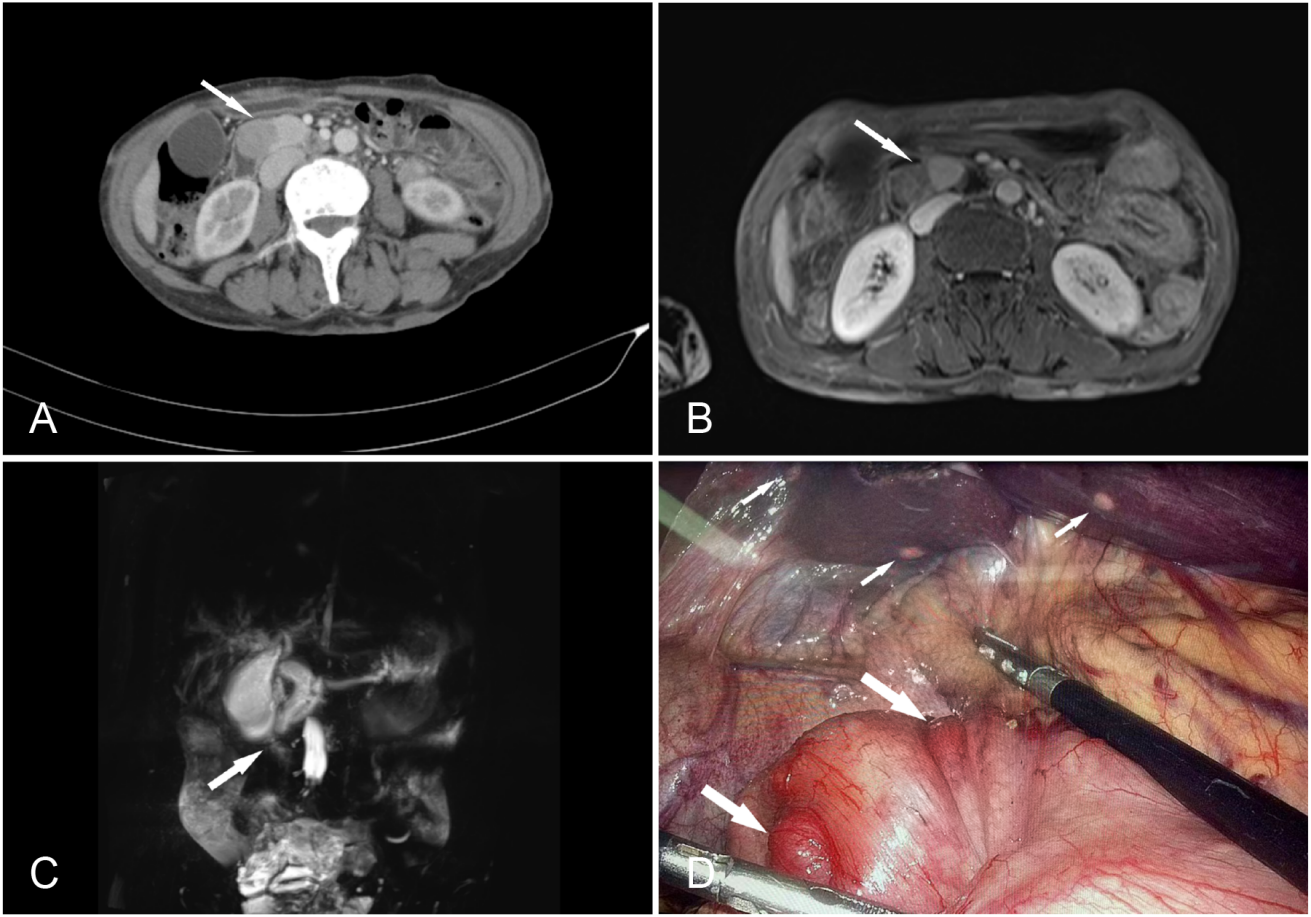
(A) Contrast-enhanced computed tomography of the abdomen showing a prominent enhancing soft tissue mass (arrow), suggesting a diagnosis of a duodenal ampulla tumor. (B) MRI venous time shows a mass at the pancreas head and ampullae area (arrow), approximately 38 mm × 20 mm × 28 mm. (C) Magnetic resonance cholangiopancreatography (MRCP) showing a significant dilation of the intra- and extrahepatic bile ducts and the main pancreatic duct, with visible truncation at the junction of the two (arrow). (D) During the operation, multiple small nodules were identified on the serosal surface of the stomach (large arrows) and multiple metastasis on the liver (small arrows).
